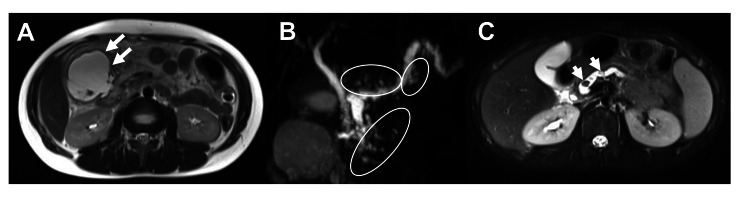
Abdominal MRI and MRCP findings Axial contrast-enhanced T1-weighted MRI (A) shows a round cystic lesion with homogenous fluid density and a well-defined wall (arrows). MRCP (B) shows numerous small cysts on the ventral side of the pancreatic body (circles). T2-weighted MRI (C) shows defects in the main pancreatic duct (arrows), as well as dilatation of the main pancreatic duct and thinning of the pancreatic parenchyma. MRI: magnetic resonance imaging; MRCP: magnetic resonance cholangiopancreatography
